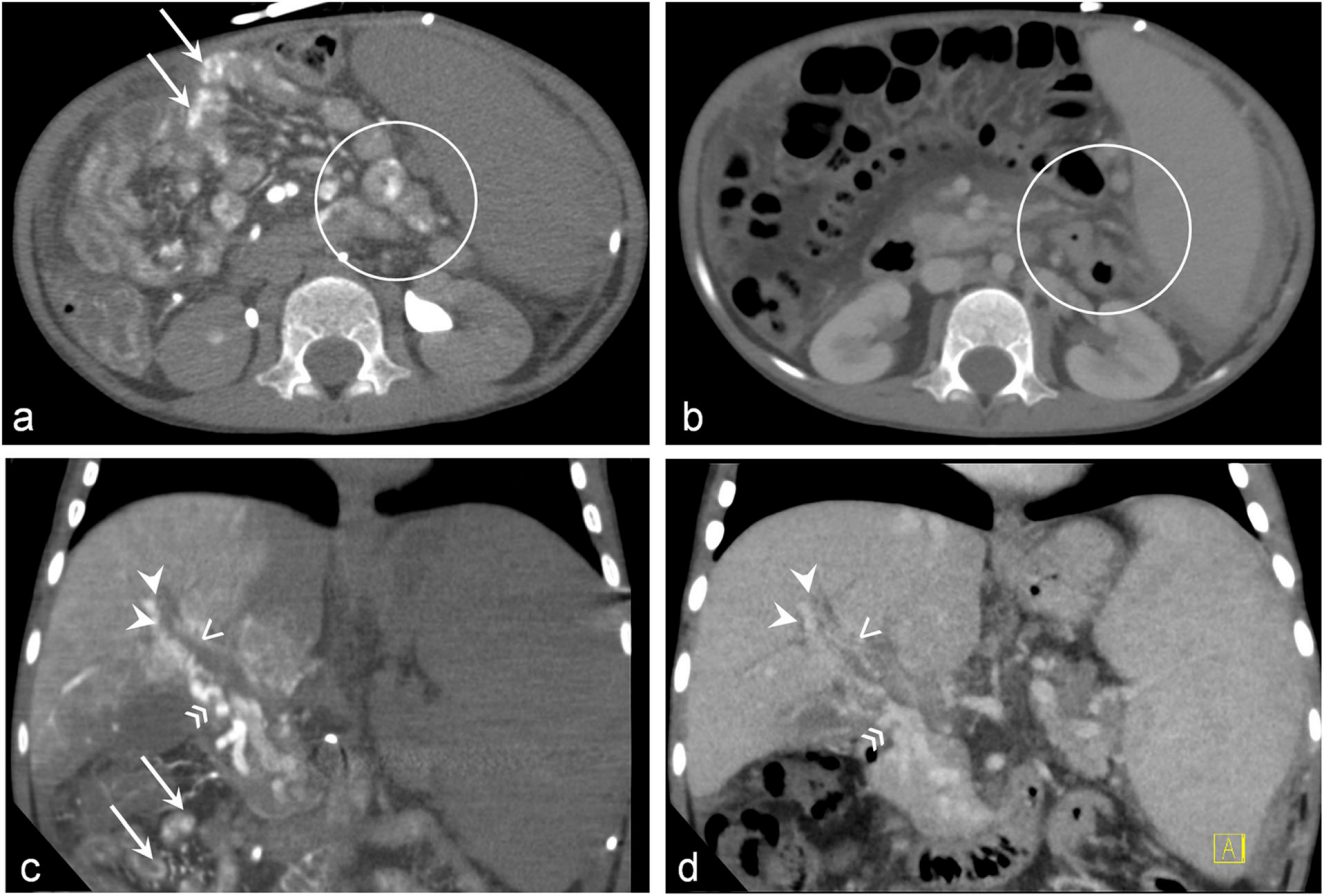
Comparison of computed tomography (CT) arterioportography (AP) (a, c) with standard CT after intravenous contrast injection (portal venous phase: b, d) in a 6-year-old male patient with portal vein thrombosis. Axial (a) and coronal (c) images of CT AP after contrast injection into the superior mesenteric artery allow to clearly detect multiple jejunal varices (arrows and circle) and portal biliopathy (arrowheads and open arrows: multiple small peribiliary collaterals, irregular and dilated bile ducts). Axial (b) and coronal (d) standard CT in the portal venous phase after intravenous contrast injection performed 2 weeks before CT AP does not detect any jejunal varices; evidence of portal biliopathy is lower than that provided by CT AP
